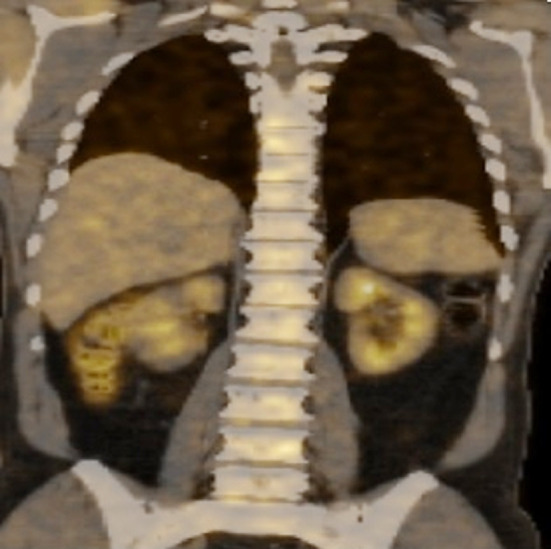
PET-CT showing low FDG avid small areas of osteolytic and patchy sclerosis involving multiple dorso lumbar vertebrae. PET: Positron emission tomography – computed tomography, FDG: Fluorodeoxyglucose
